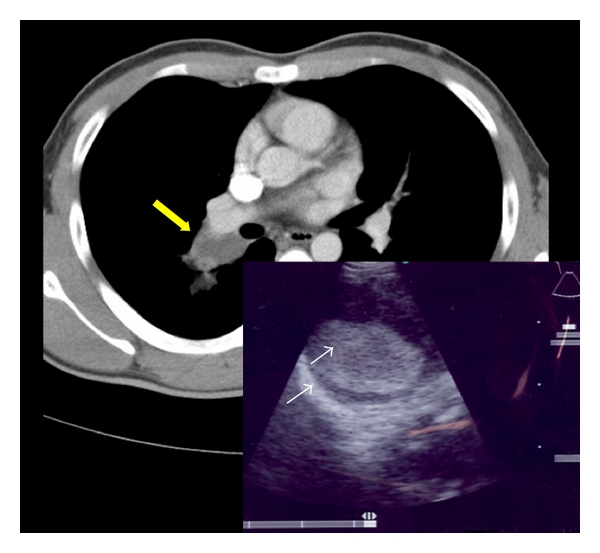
CT scan showing a soft tissue lesion at the right hilum (yellow arrow). Insert image: EBUS image showing a round lesion with an echogenic center and thickened hyperechoic walls (white arrows).
